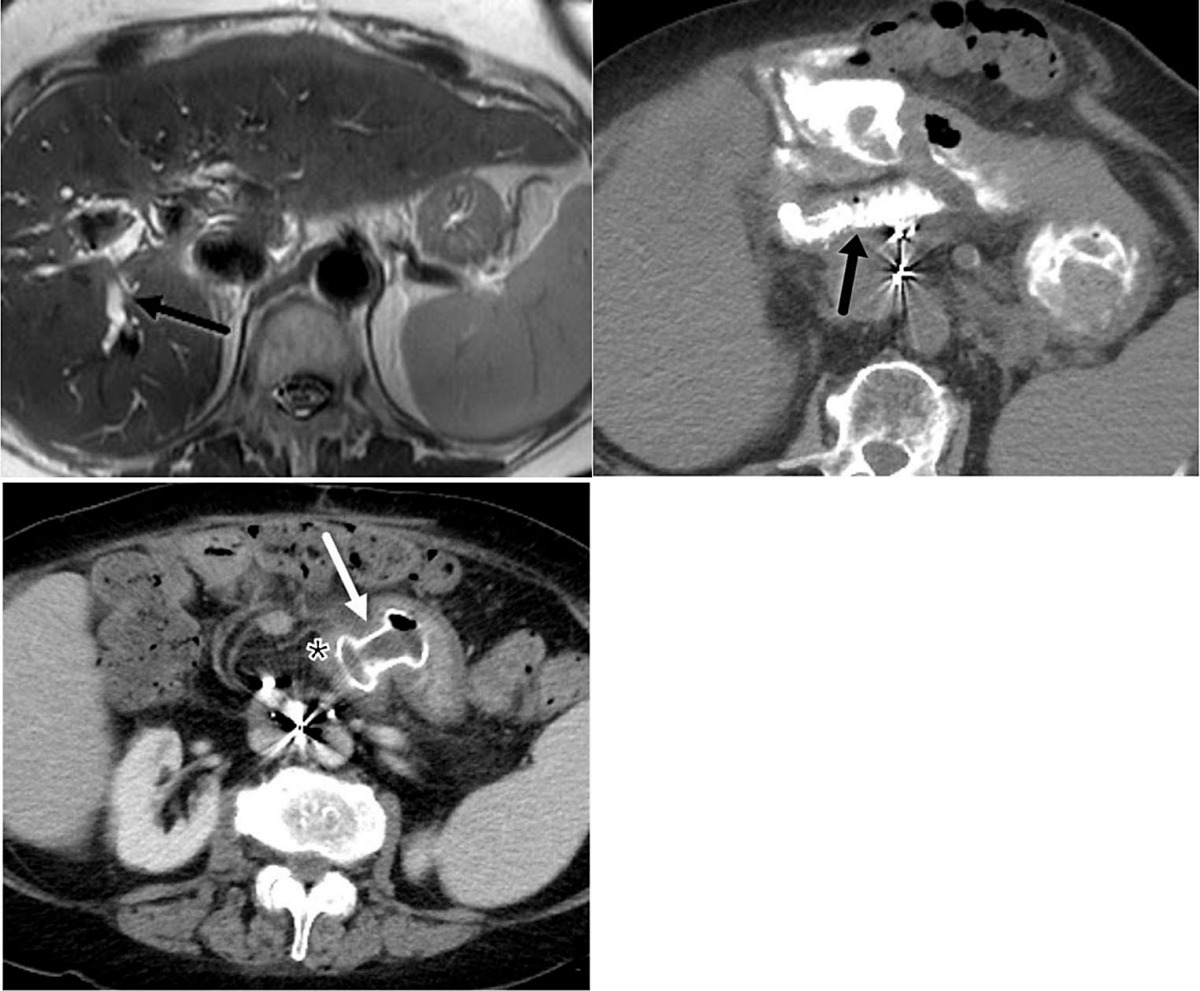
76 year-old female with history of Whipple surgery for malignant IPMN developed biliary strictures after chemotherapy and radiation therapy. The patient required technically challenging ERCPs for biliary stricture dilatation every several months due to the recurrent nature of biliary strictures and recurrent cholangitis. A. Axial T2-weighted MRI shows intrahepatic biliary dilatation (black arrow) requiring repeated stricture dilatations and frequent stent exchanges every 2–3 three months. Frequent ERCPs were technically challenging due to surgically altered anatomy after Whipple surgery. A proposed intervention included creating a permanent conduit traversing the gastrojejunostomy between the remnant stomach and the pancreaticobiliary limb to allow ease of endoscopic access for biliary dilatation/drainage. B. To promote placement of LAMS across the gastrojejunostomy, the pancreaticobiliary limb had to be distended prior to LAMS deployment by contrast introduced via percutaneous transhepatic cholangiogram through hepaticojejunostomy. This axial CT image demonstrates distention of the pancreaticobiliary limb with contrast (black arrow). C. Contrast-enhanced axial CT confirms that the LAMS (white arrow) was successfully placed between the stomach and pancreaticobiliary limb (asterisk) to create a permanent indwelling conduit for ERCP access
